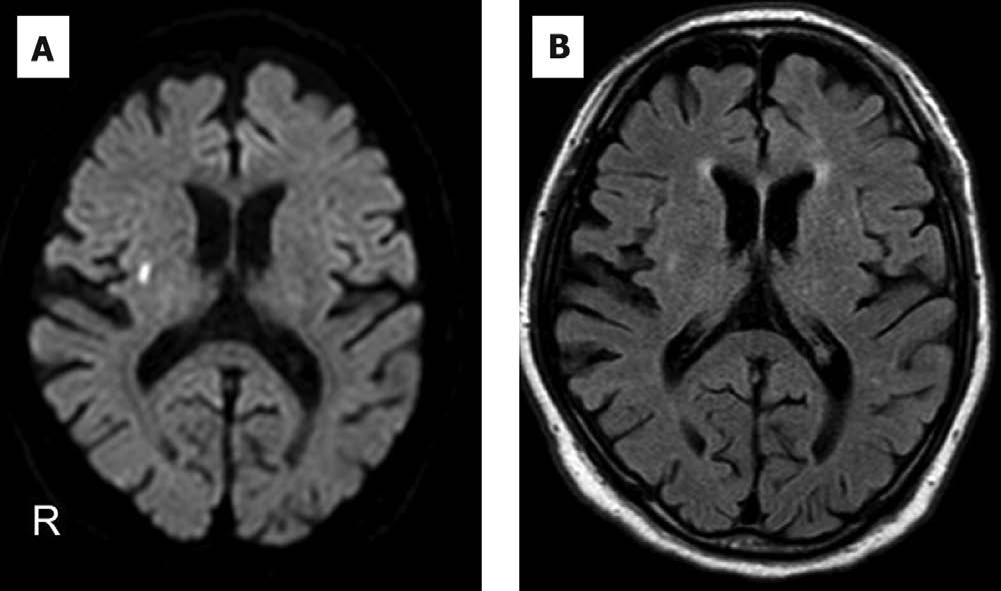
Brain MRI in Case 6 admitted with delayed encephalopathy (A, diffusion-weighted image; B, fluid-attenuated inversion recovery image). Acute lacunar infarction is seen in the right basal ganglia. MRI = magnetic resonance imaging.
